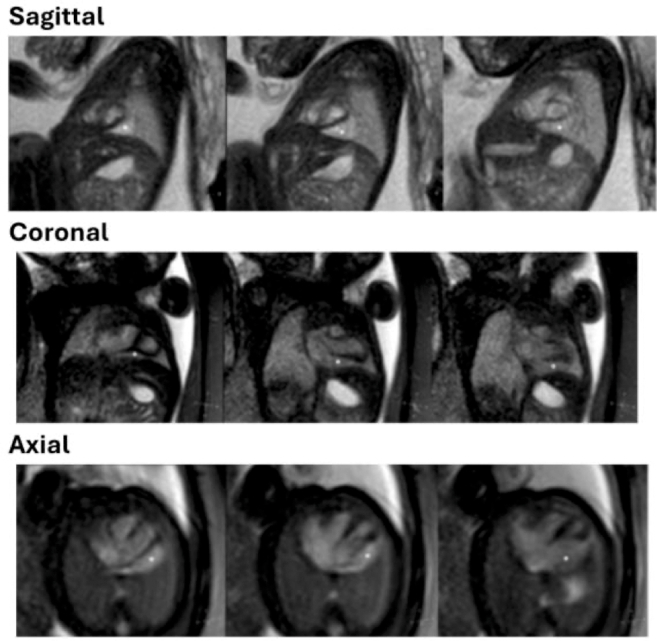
Fetal Magnetic Resonance Imaging at 29 Weeks Demonstrating a Well-Circumscribed Cystic Structure (Asterisk) Along the Left Posterior Atrioventricular Groove Extending Inferiorly Along the Thoracic Surface of the Diaphragm on Sagittal, Coronal, and Axial Sequences
